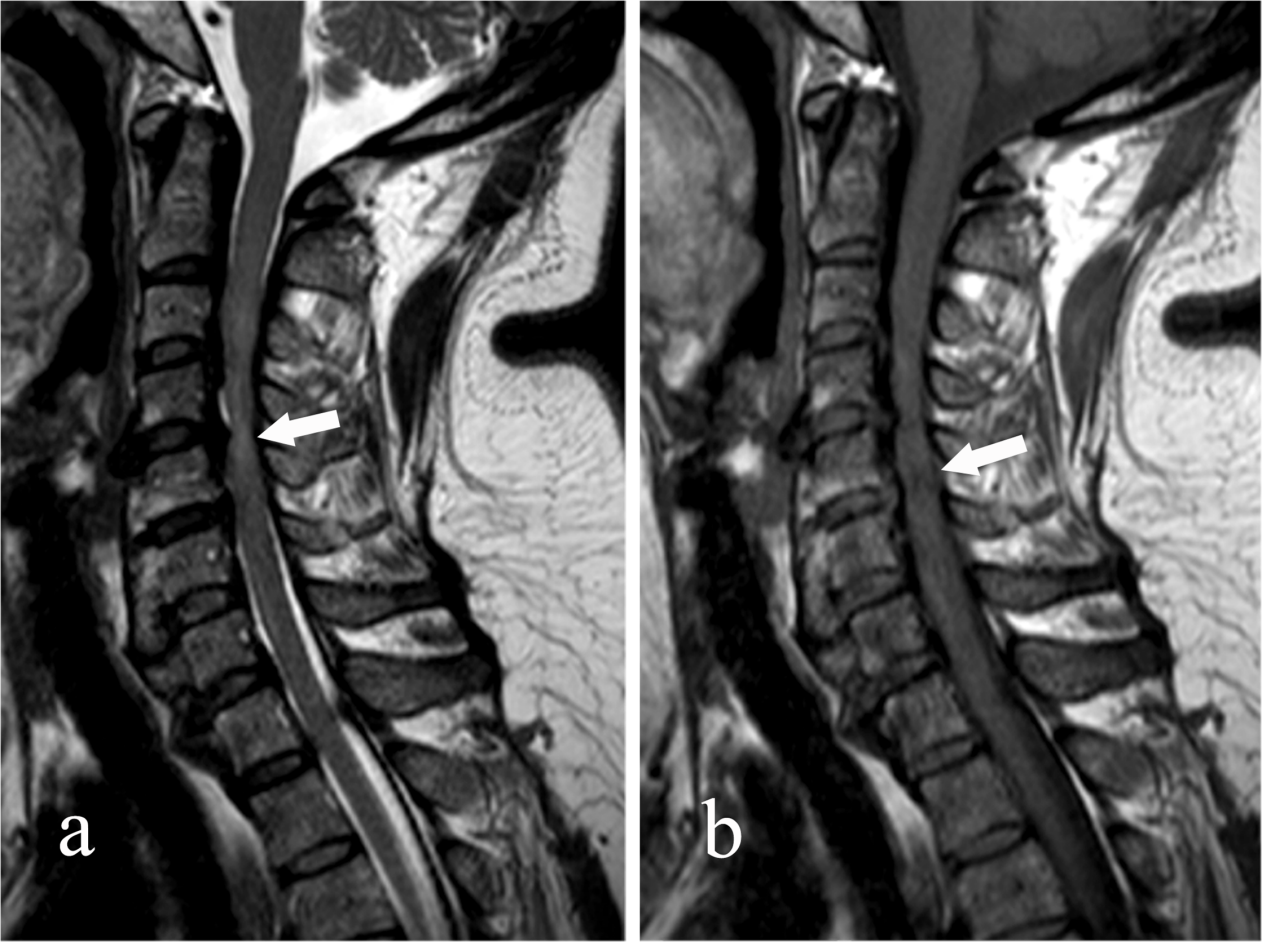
Intramedullary changes in signal intensity on both T1-weighted imaging (T1WI) and T2-weighted imaging (T2WI) of MRI were assessed. Fig 7a shows a T2 hyper-intensity intramedullary change (arrow) and Fig 7b shows a T1 hypo-intensity intramedullary change (arrow) on saggital MRI scan.
